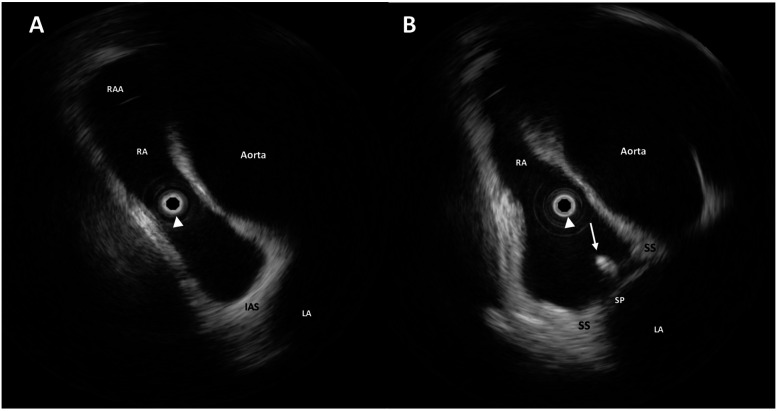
Intraprocedural rotational intracardiac echocardiography (rICE) using a 9 F–9 MHz Ultra ICE catheter-based ultrasound probe located at the center of the images (white arrowhead) in the aortic valve axial plane showing the enlargement of the aortic root and the ascending aorta shrinking the right atrium (A). The guidewire (white arrow) crossing the interatrial septum through the PFO (B). RAA, right atrial appendage; RA, right atrium; LA, left atrium; SS, septum secundum; SP, septum primum.
